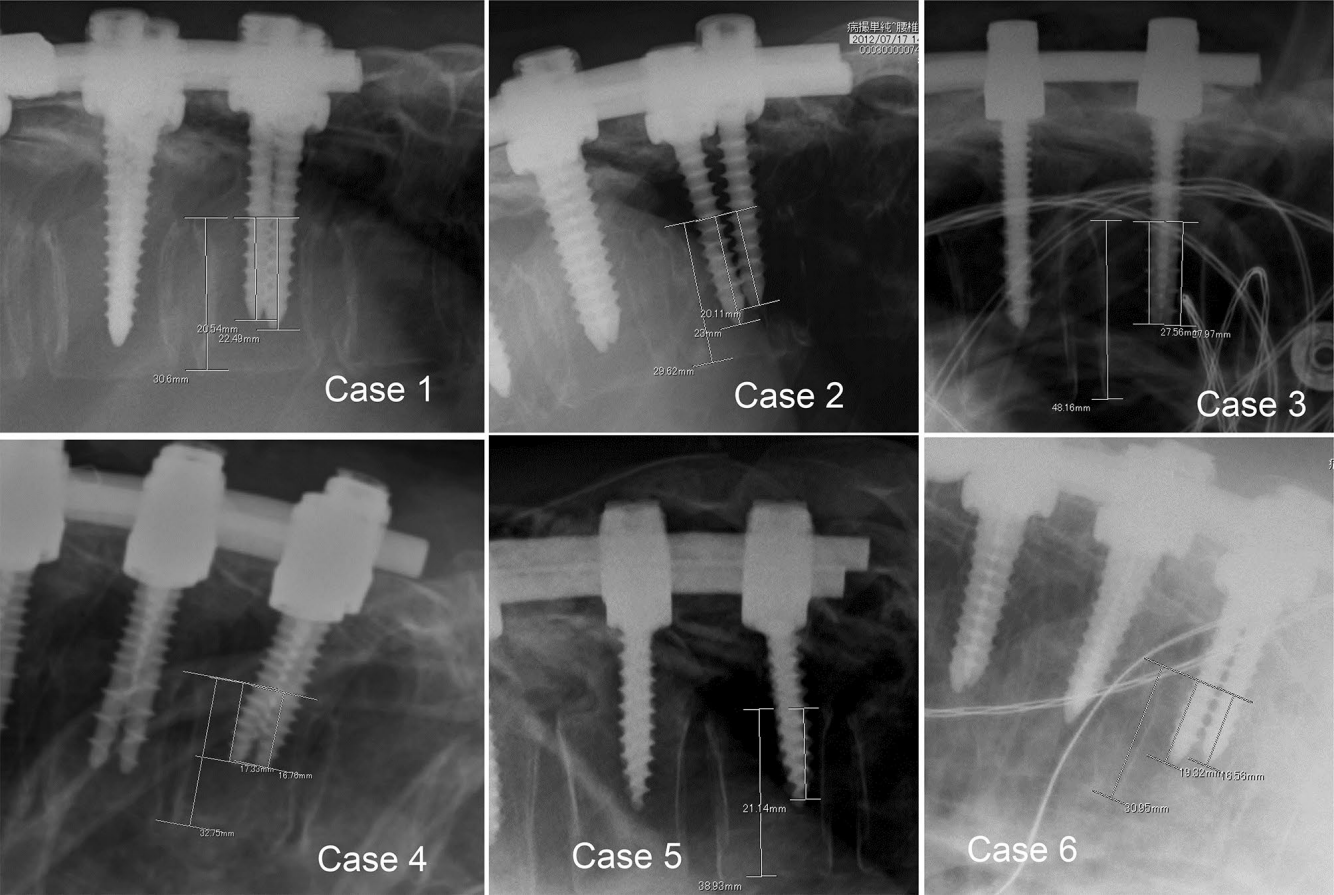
Radiographs in cases with paraplegia due to UIV fracture. All cases had ORPS < 73% (Case 1: 70.3%, Case 2: 72.8%, Case 3: 57.7%, Case 4: 52.0%, Case 5: 54.2%, Case 6: 63.9%). *ORPS* occupancy rate of pedicle screw, *UIV* upper instrumented vertebra.
